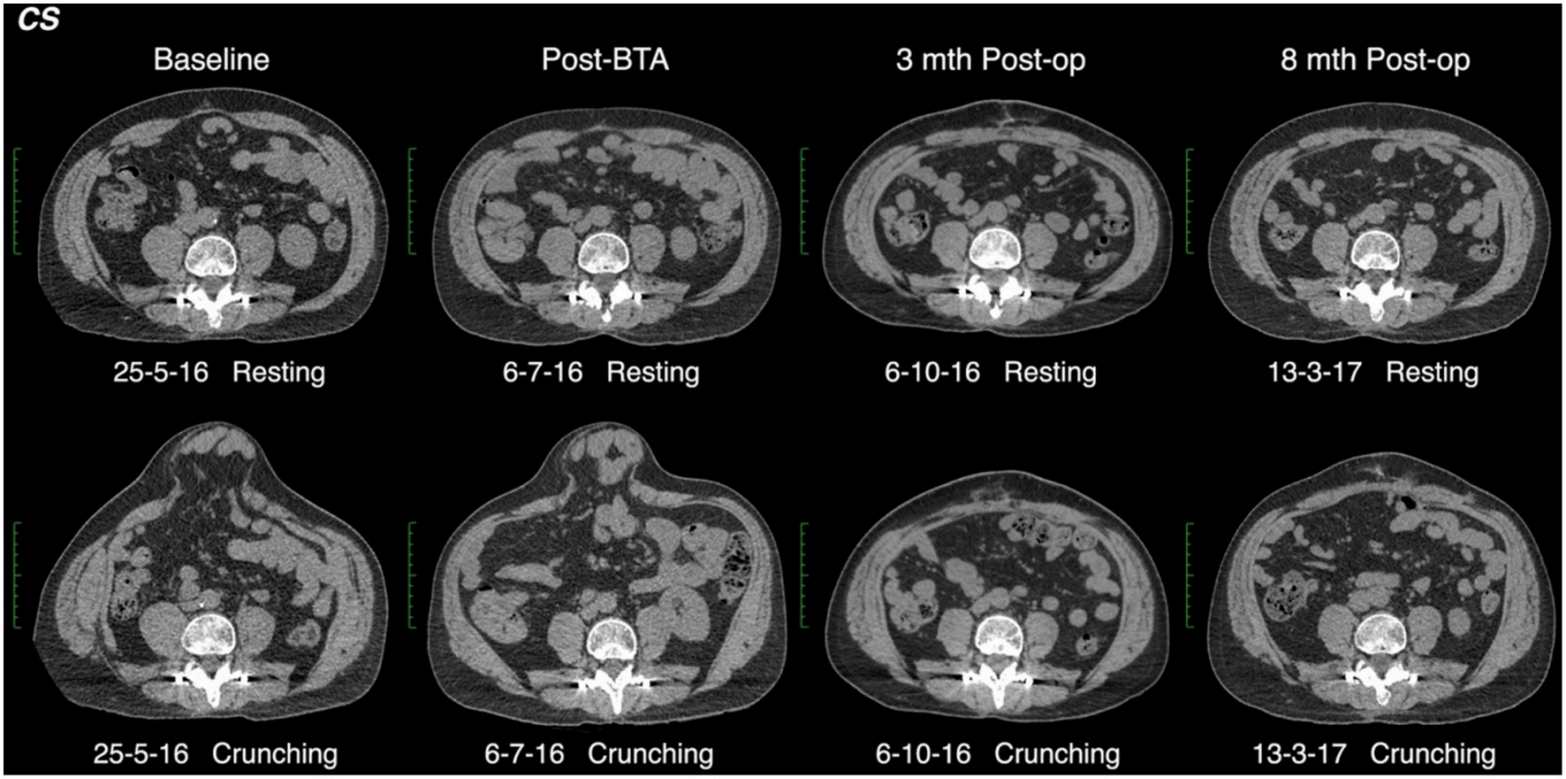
Functional CT methodology (patient #11). Comparison resting and crunching images obtained at the same axial level were used to measure the contractility index for each muscle segment.
